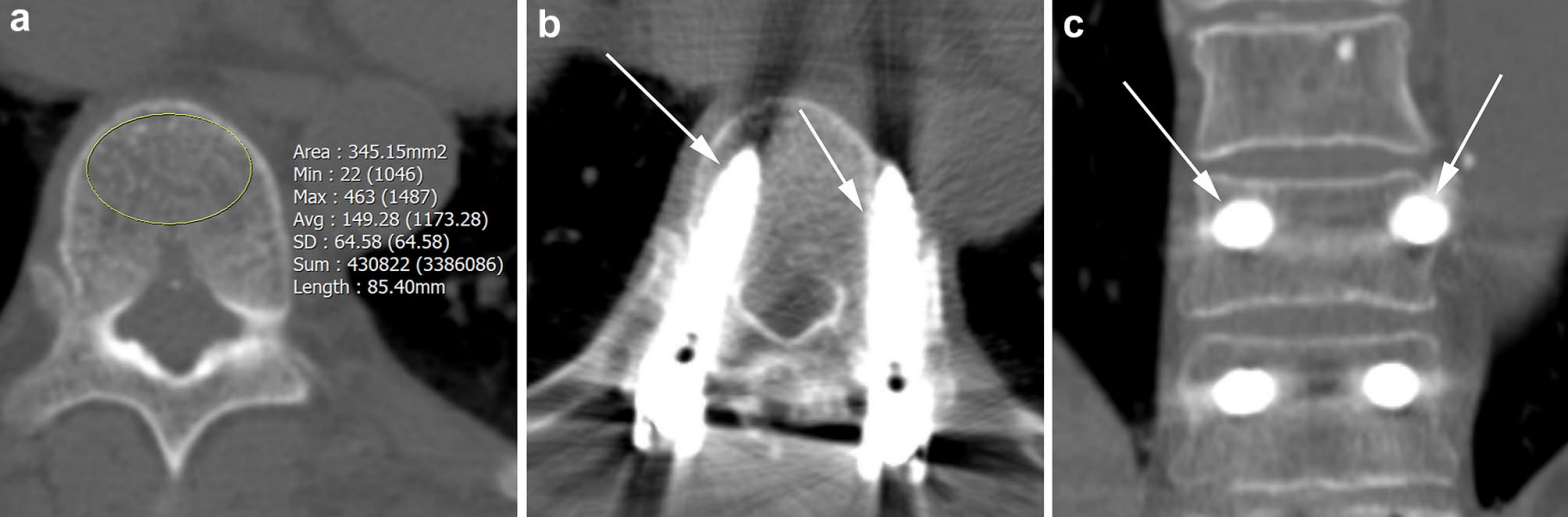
A 77-year-old woman who underwent spinal fusion surgery from T10 to S1. In the axial plane of the preoperative CT scan (a), the Hounsfield unit value of the upper instrumented vertebral body (T10) was 149.28, which was higher than the cutoff value. Postoperative CT performed 2 years and 7 months after surgery did not show screw loosening in the axial (b) or coronal (c) planes (arrows). *min* minimum,*max* maximum, *avg* average, *SD* standard deviation.
